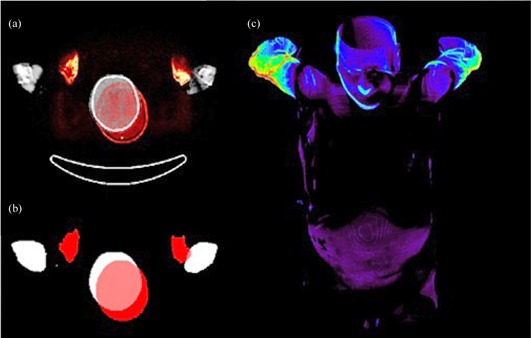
The misalignment of the arms and head is demonstrated on the overlaid CT and MRI images (a). The same images are shown (b) after segmentation and labeling (MR, red; CT, white). 3D view of distance map for whole body for the worst case study (c).
